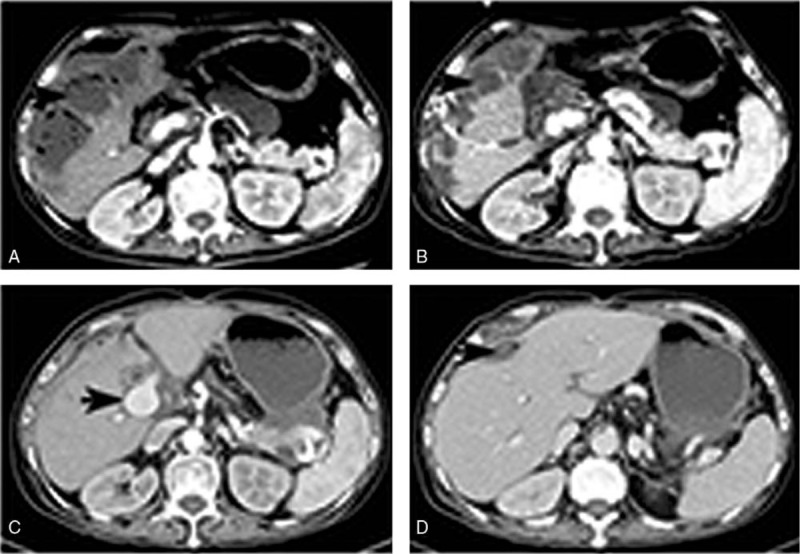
liver recipient (recipient 1). (A) Enhanced total abdomen CT scan revealed the thrombosis of hepatic artery and massive liver necrosis 14 days after liver transplantation. (B) Reduction in sizes of liver abscesses after percutaneous peritoneal drainage 2 months following liver transplantation. (C) Significant reduction in sizes of liver abscess 7 months after liver transplantation. (D) Enlarged portal vein and numerous branches 7 months after liver transplantation. CT = computed tomography.
